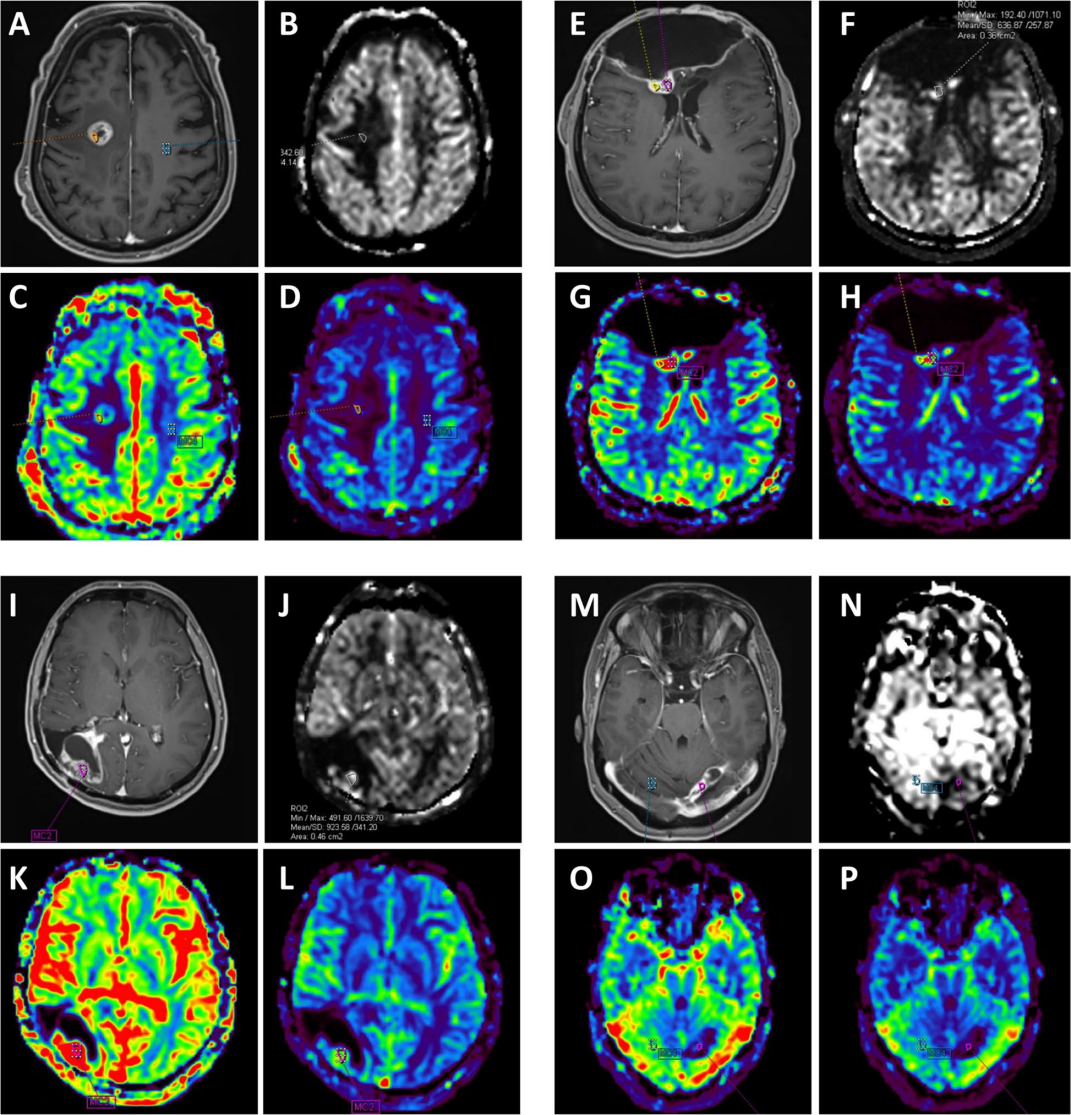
Post-RT evaluation in a patient with lung cancer and brain metastasis; A Contrast-enhanced fat-suppressed T1: metastatic lesion showing contrast enhancement, B ASL CBF: minimal signal change in the lesion site, C DSC CBV: Significant hyperperfusion in the lesion site, D DSC CBF: Mild moderate hyperperfusion is observed compared to CBV. It is categorized as RRT with follow-up imaging and clinic. Control image with recurrence of leiomyosarcoma metastasis and after surgery and RT history, E Contrast-enhanced fat-suppressed T1: Solid lesion with contrast enhancement in the posterior part of the operation cavity wall, F ASL CBF: Hyperperfusion in the lesion site, G DSC CBV: Marked hyperperfusion in the lesion site, H DSC CBF: Hyperperfusion in the lesion site. It is categorized as RRT with follow-up imaging and clinic. Case with a history of surgery and RT due to Grade 3 Astrocytoma, I Contrast-enhanced fat-suppressed T1: Solid-looking area showing contrast enhancement on the operation cavity wall, J ASL CBF: Hyperperfusion in the contrasted area, K DSC CBV: Significant hyperperfusion in the contrasted area, L Hyperperfusion is observed in the contrasted area. It is categorized as RRT with follow-up imaging and clinic. Control MRI in the case with a history of surgery and RT due to breast cancer brain metastasis, M Contrast-enhanced fat-suppressed T1: Moderate thickening of the wall medial to the operation cavity is observed in the left cerebellar hemisphere, N ASL CBF, O DSC CBV, P DSC CBF: The part medial to the cavity is hypoperfused compared to the opposite hemisphere. When evaluated with follow-up findings, it is in favor of TCR
